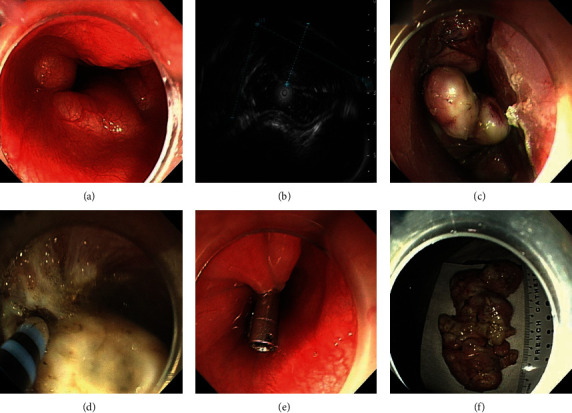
Submucosal tunneling endoscopic resection treatment for a gastric GIST: (a) gastric GIST identified by endoscopy; (b) the same gastric GIST observed by endoscopic ultrasound; (c, d) resection of the tumor via submucosal tunneling; (e) close the wound; (f) the resected tumor. GIST: gastrointestinal stromal tumor.
